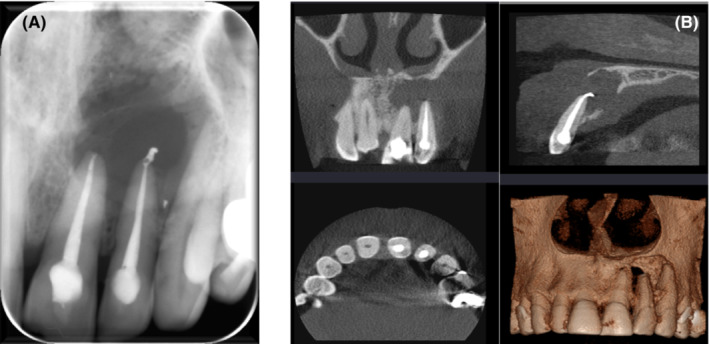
Preoperative radiograph (A) Periapical Radiograph, (B) The lesion on CBCT.
